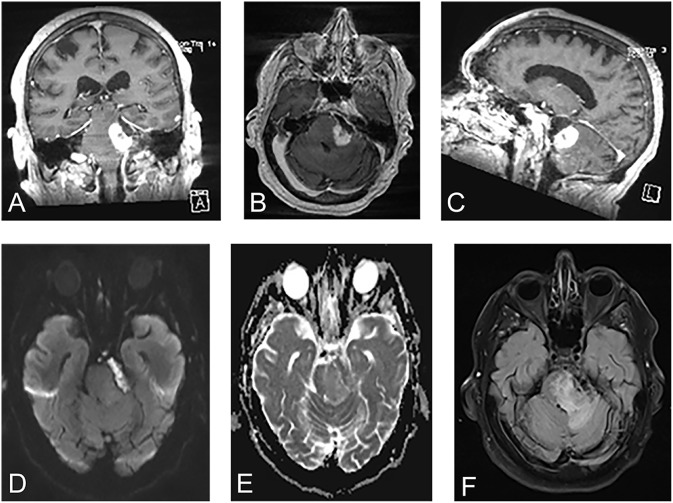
Pre-operative magnetic resonance images revealed a cystic lesion that was T1 hypointense, T2 hyperintense, and peripherally enhancing within the left cerebellopontine angle wrapping around the lateral brainstem. Adjacent to the cystic lesion was a T1 isointense, T2 hyperintense, avidly enhancing infiltrative component involving the brainstem, cerebellar peduncle, and cerebellum. (A) Coronal T1-weighted contrast enhanced. (B) Axial T1-weighted contrast enhanced. (C) Sagittal T1-weighted contrast enhanced. (D) Diffusion weighted-imaging. (E) Apparent diffusion coefficient. (F) Fluid-level attenuated inversion recovery.
